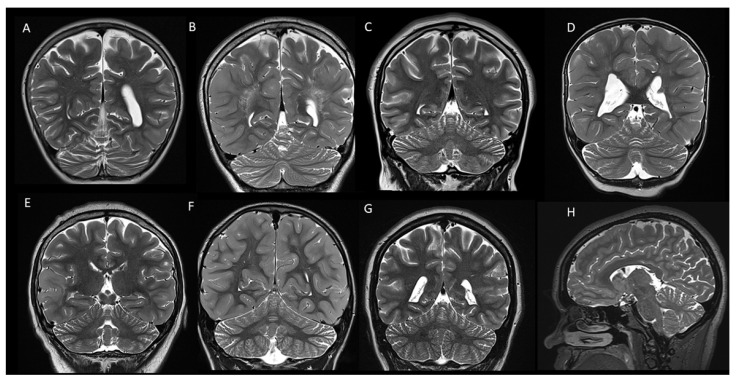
Coronal (A–G) and sagittal (H) T2-weighted images of 8 patients with tuberous sclerosis demonstrating mild diffuse cerebellar atrophy with widening of the cerebellar fissures.
